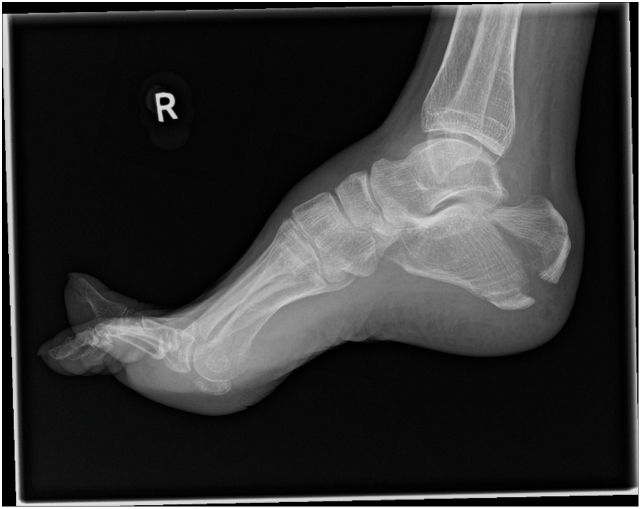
Lateral right foot radiograph demonstrates a displaced ‘beak’ calcaneal tuberosity avulsion fracture.
